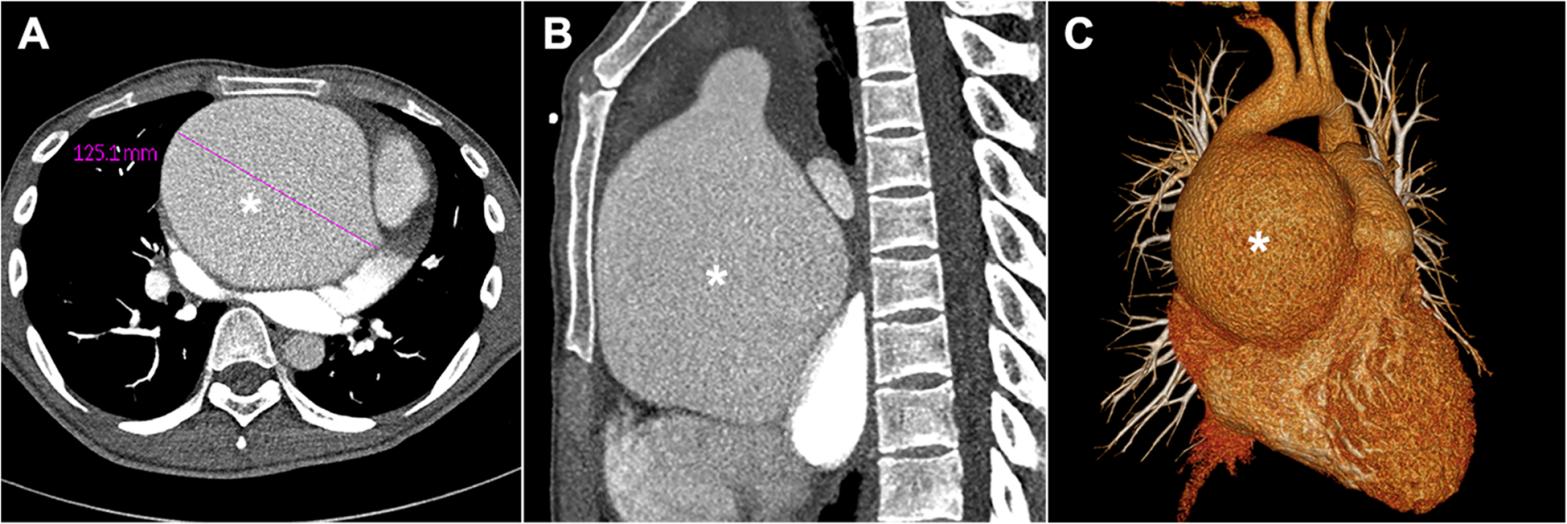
Results of preoperative imaging examinations: a and b Transverse view and sagittal view of enhanced thoracic computed tomography scan demonstrated the size of the aneurysm, respectively; c Three-dimensional enhanced computed tomography scan showed that the aortic root aneurysm is like a “bulging balloon”; *, aortic root aneurysm
